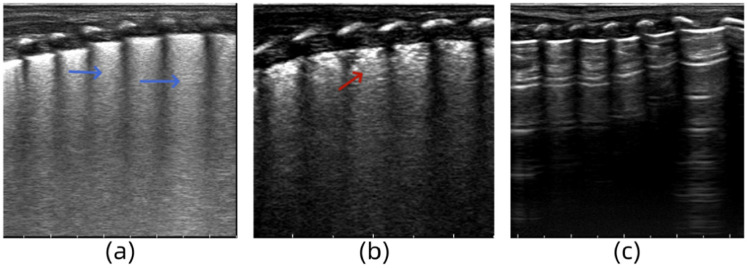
(a) Lung ultrasonography (LUS) reveals dense B-lines in lung fields. (b) LUS reveals a small number of B-lines in the intercostal spaces of the upper lung field and AIS manifestation in the lower lung field. (c) The LUS findings return to normal.
